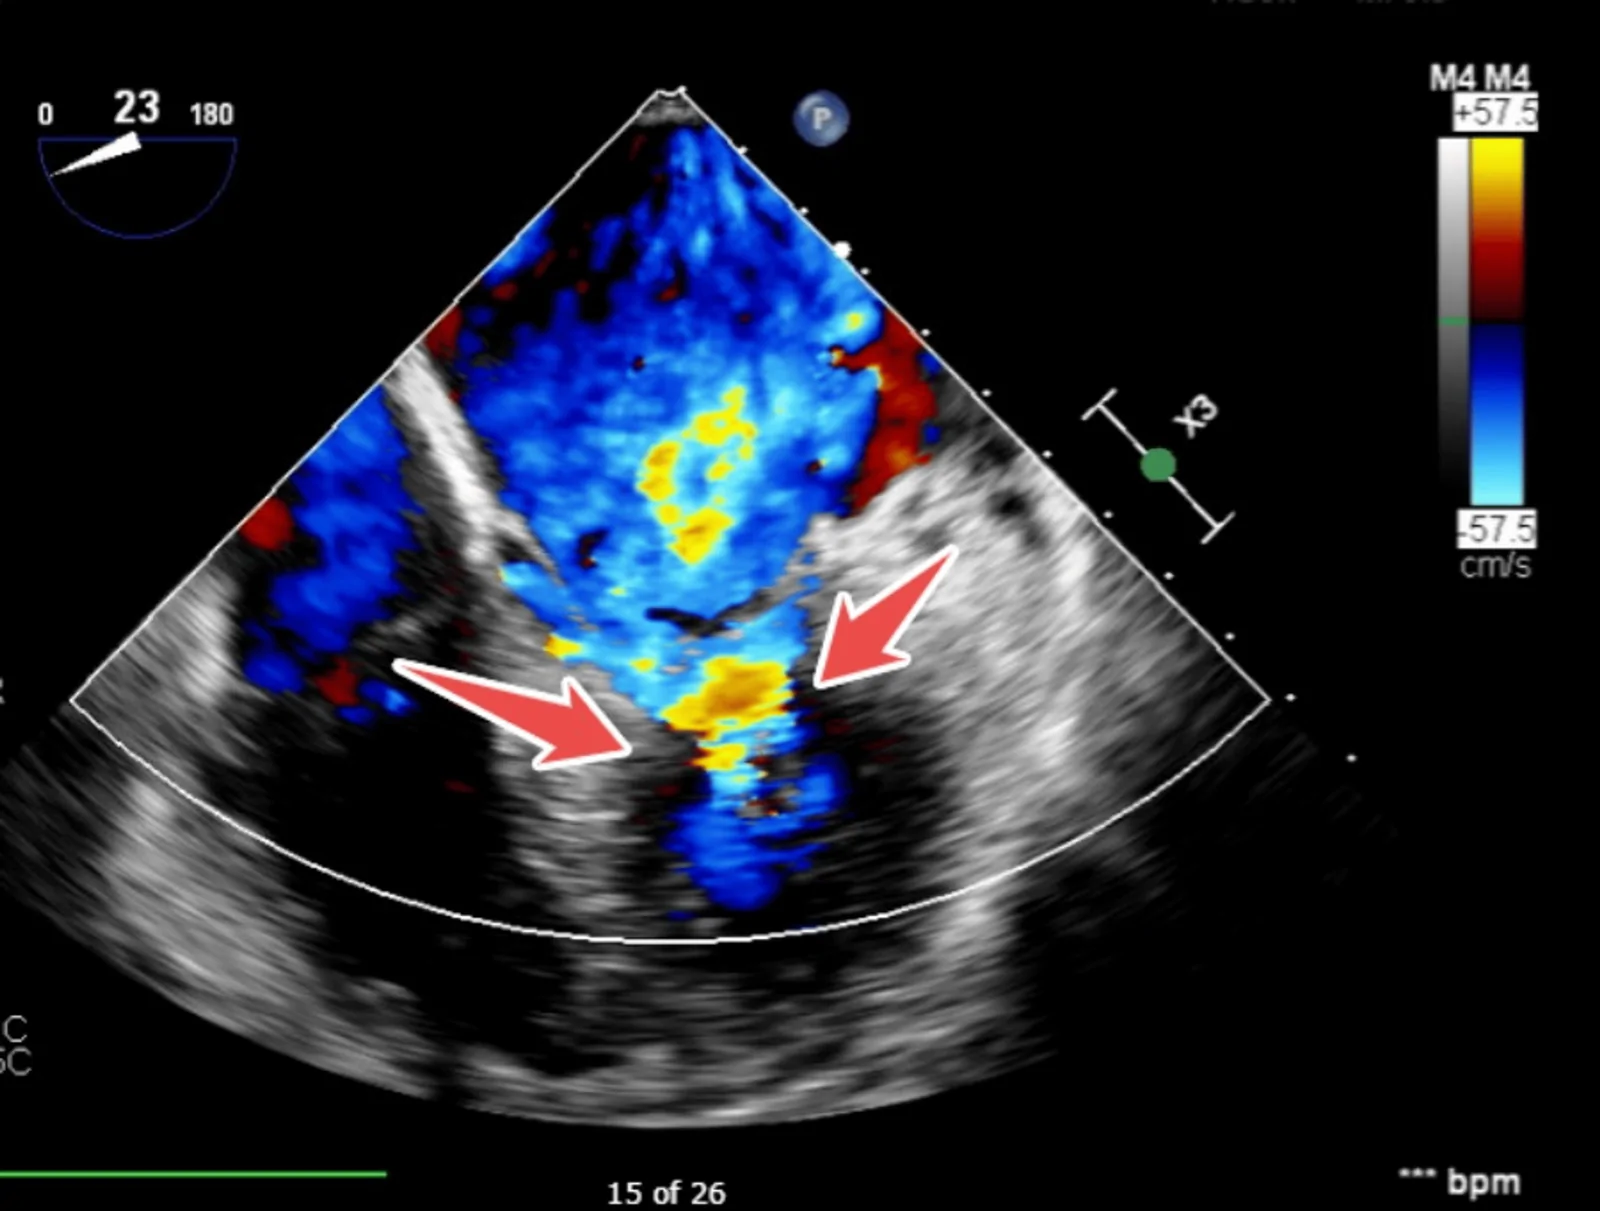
Transesophageal echocardiographic assessment demonstrating severe mitral regurgitation highlighted by arrow.
